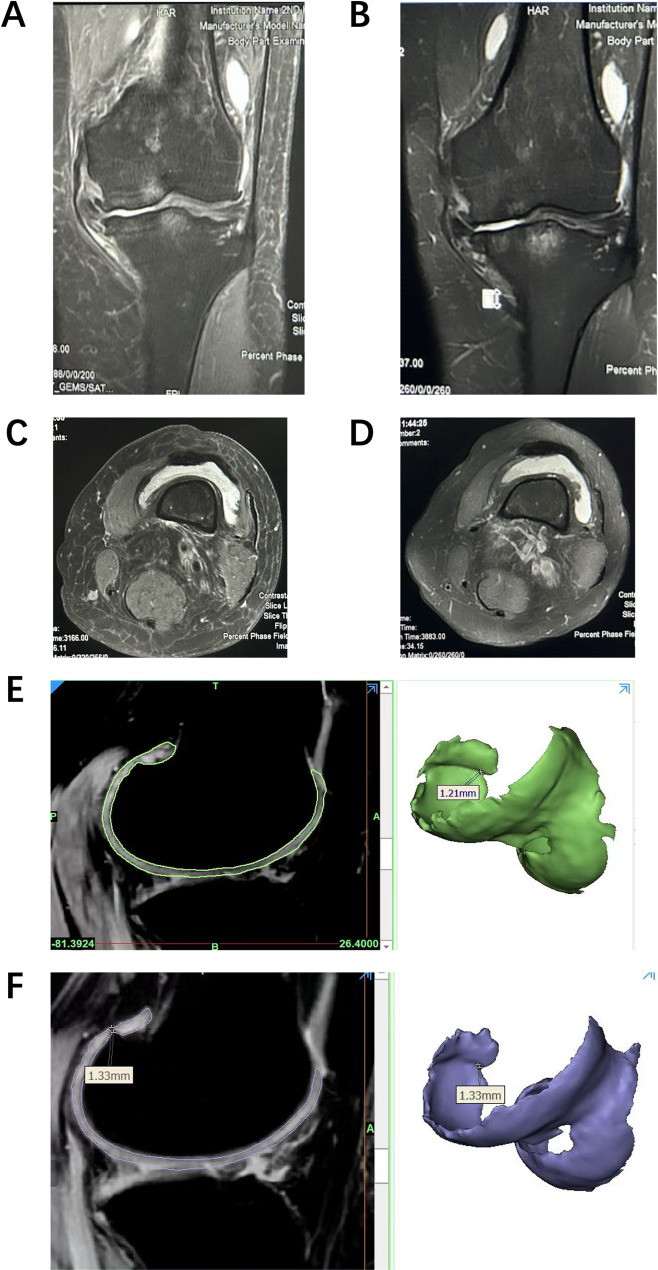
Knee joint MRI before and after hUC-MSCs-Exos injection: (a) Pre-injection knee MRI showing extensive bone marrow edema. (b) Nine months after injection, knee MRI shows reduced bone marrow edema area. (c) Pre-injection knee MRI showing a large volume of joint effusion. (d) Post-injection knee MRI showing a smaller volume of joint effusion. (e) Pre-injection knee MRI showing significant damage to the femoral cartilage. (f) Post-injection knee MRI showing thickened cartilage at the same location of the femoral condyle
